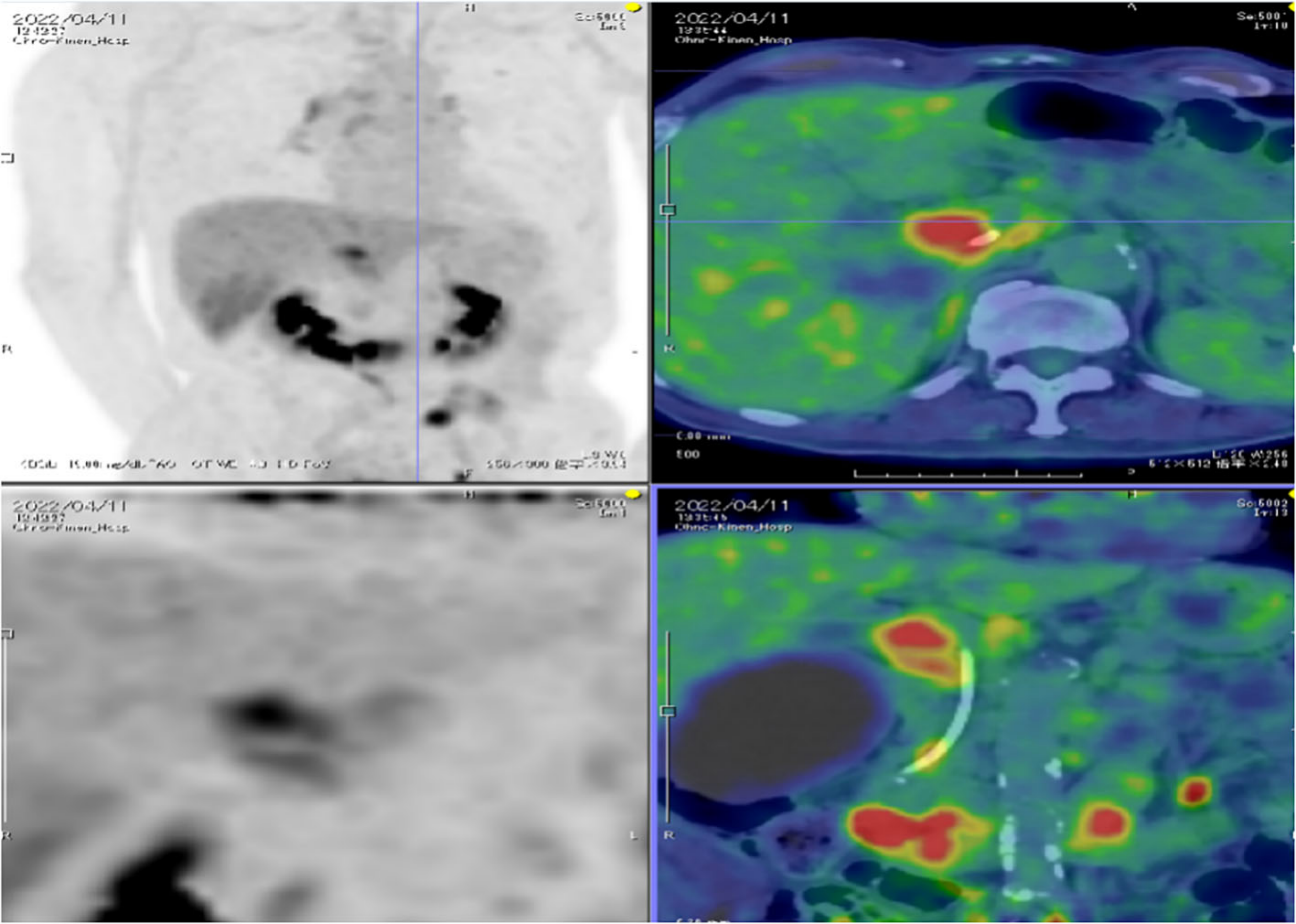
The patient’s positron emission tomography-computed tomography (PET-CT) performed prior to proton therapy, exhibiting a hypermetabolic focus at the hepatic portal with associated retroperitoneal lymph node metastasis, as well as the indwelling biliary stent.
